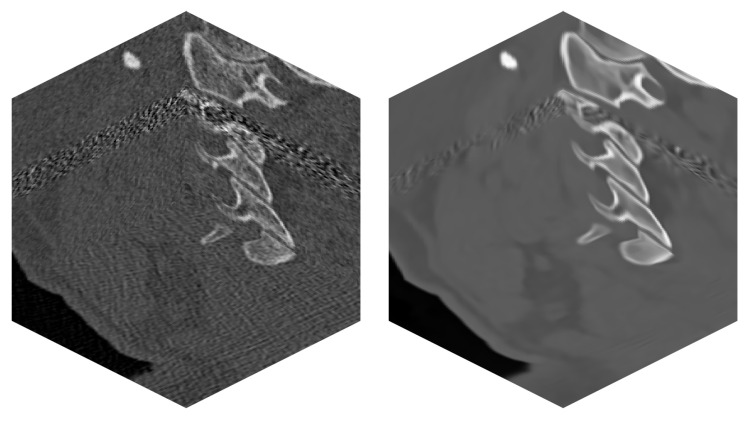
Real CT data of a patient—series of 170 images. (left)—Original noisy data. (right)—Filtered image data by BM4D.
